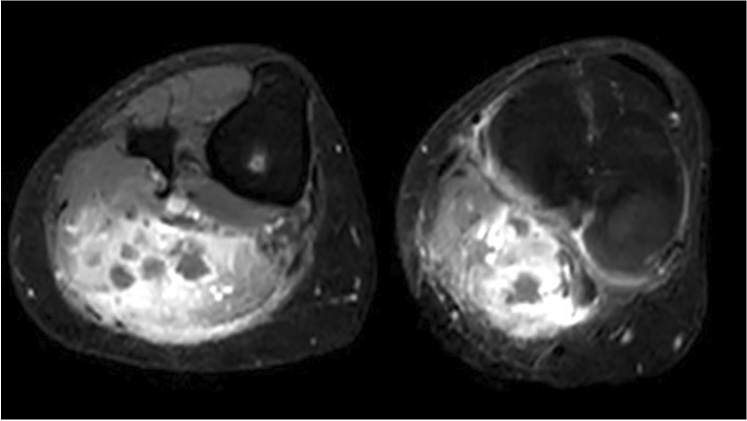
T2-weighted magnetic resonance imaging (MRI) showing edema of the gastrocnemius muscle with fluid collections and peripheral contrast enhancement. Images: Department of Diagnostic and Interventional Radiology, University Hospital Hamburg Eppendorf
